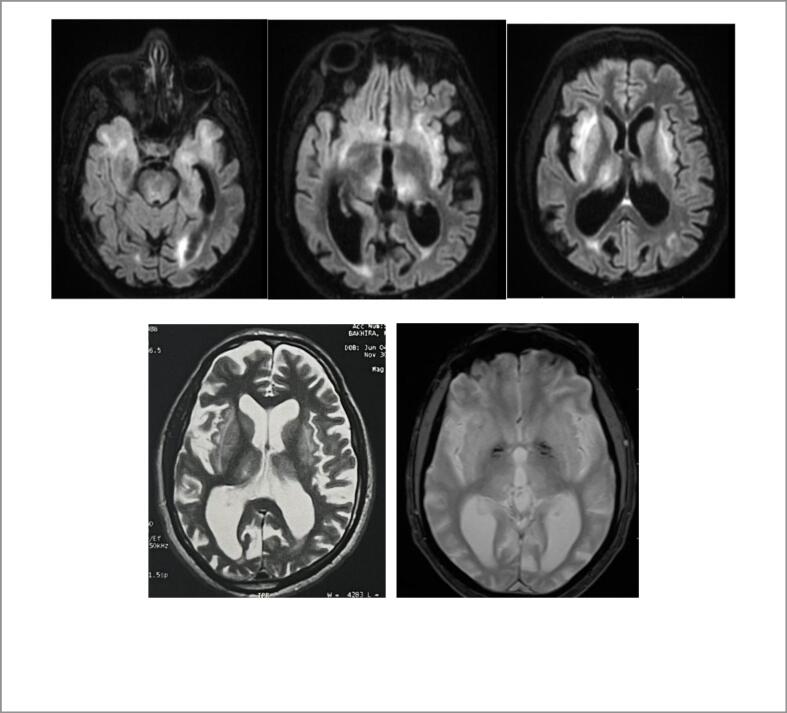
Pre-treatment brain MRI (T2-FLAIR) shows lesions in the insular-temporo-occipital lobes, and lesions in the caudate nucleus, thalamus and the pons. T2* reveals calcifications in the lentiform nucleus.
